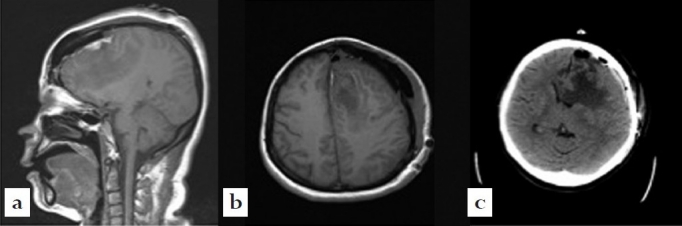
Post-operative imaging of the brain. (a) Sagittal view magnetic resonance imaging (MRI) (T1 weighted), (b) axial view MRI (T1 weighted), (c) axial view computer tomography. Imaging demonstrated gross total resection of the mass
